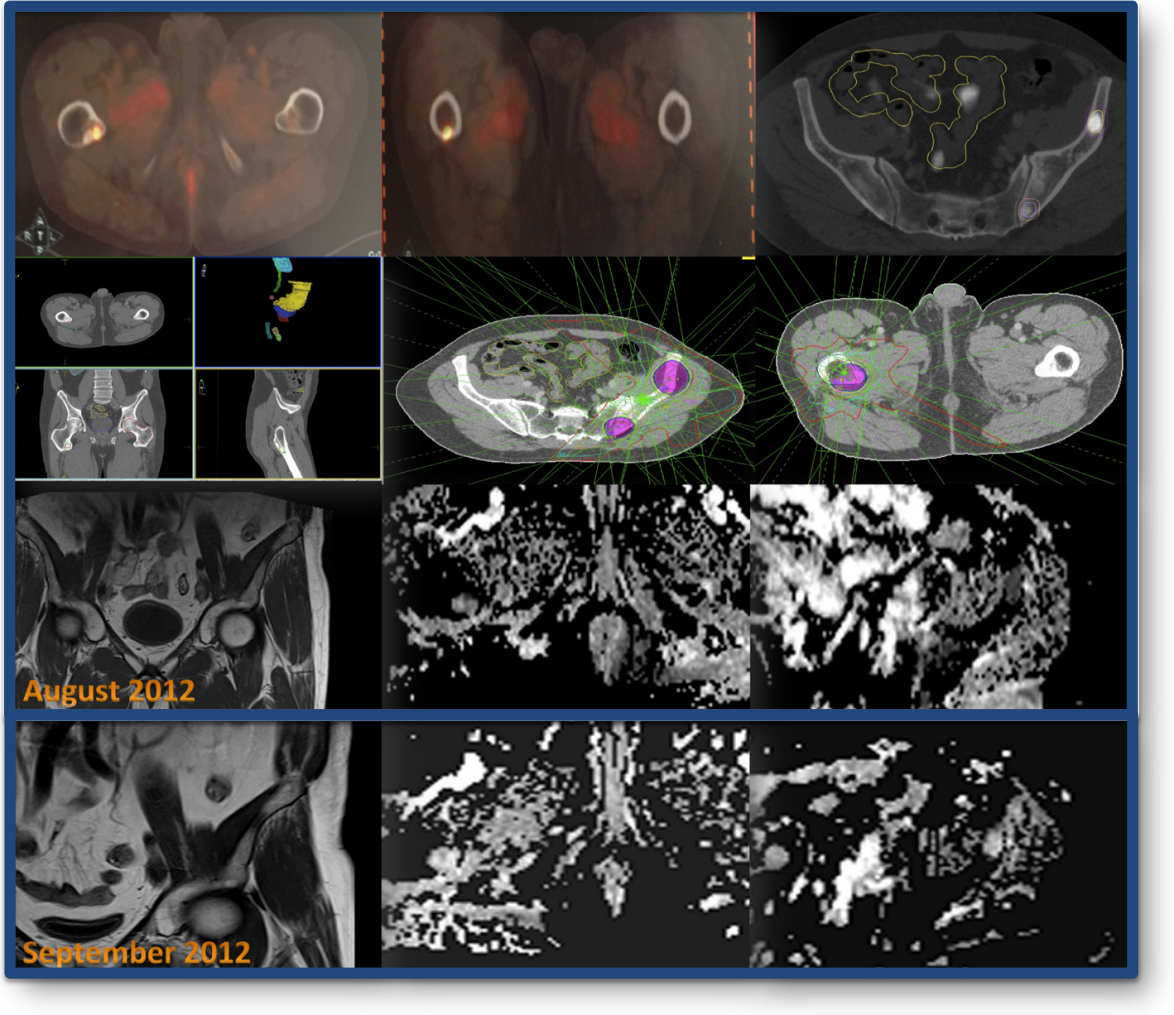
Case report: Patient treated with SBRT and evaluation of response to the changing pattern of ADC in MRI. Patient diagnosed of 3 bone metastasesof prostate cancer by PET/CT Fluror-Choline and diffusion MRI treated with SBRT (3 fractions of 10 Gy). Assessment by MRI diffusion technique, suggestiveof response to treatment.
